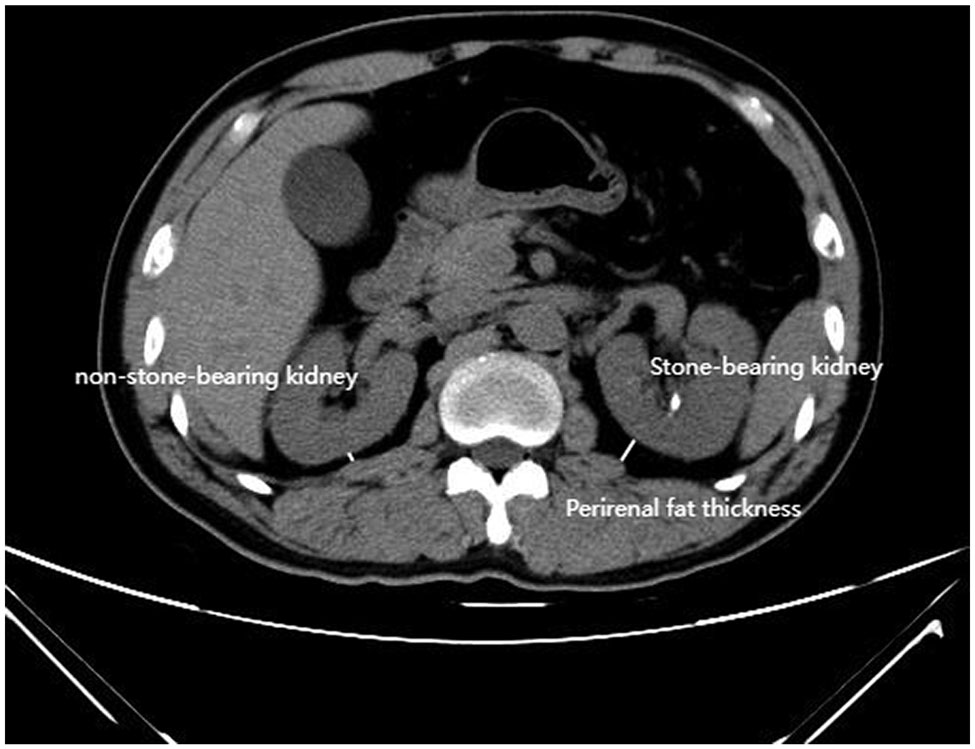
Axial CT scans at the level of renal hilum used for evaluating the perirenal fat thickness.
